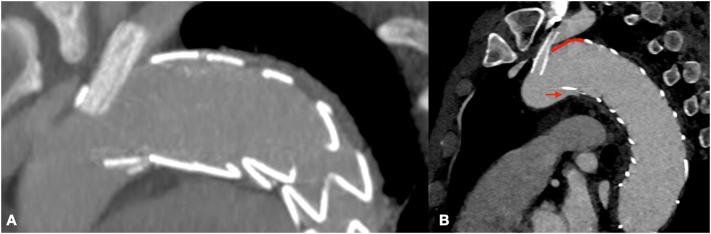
Post-operative CT-angiography after the first procedure (A) and Follow-up at 12 months showing migration of prosthesis and penetrating aortic ulcer (B).
